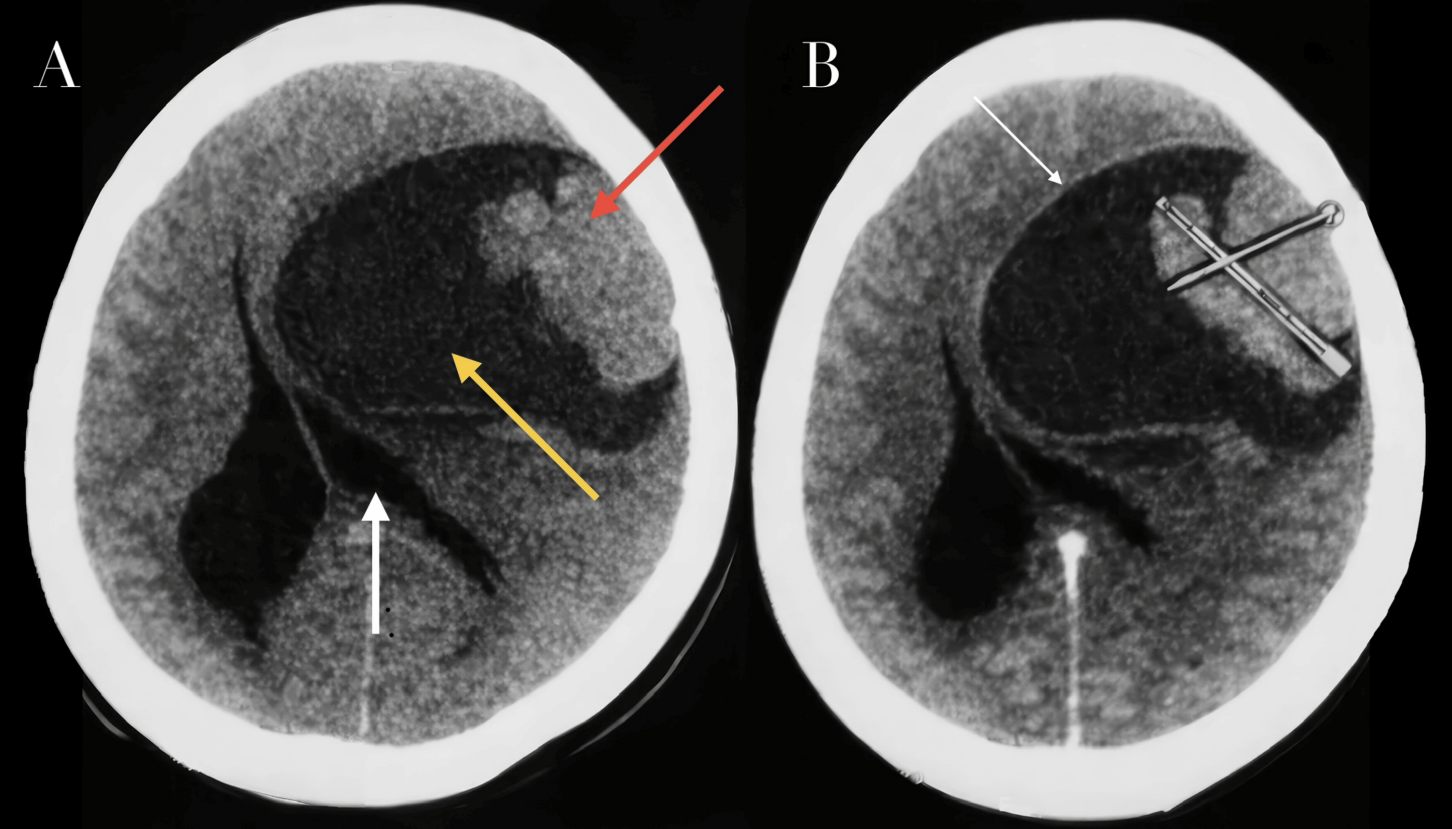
Axial non-contrast brain CT (A) and contrast CT (B) images demonstrate a well-defined extra-axial mass along the cerebral convexity. (A) Non-contrast axial CT demonstrates a large extra-axial lesion with both solid (red arrow) and cystic (yellow arrow) components, causing significant mass effect, with midline shift and near-complete compression of the ipsilateral lateral ventricle (white arrow). (B) Contrast-enhanced axial CT shows marked enhancement of the solid component, and enhancement of the cyst wall (white arrow), further supporting the diagnosis of a dural-based, extra-axial tumor. CT, computed tomography
